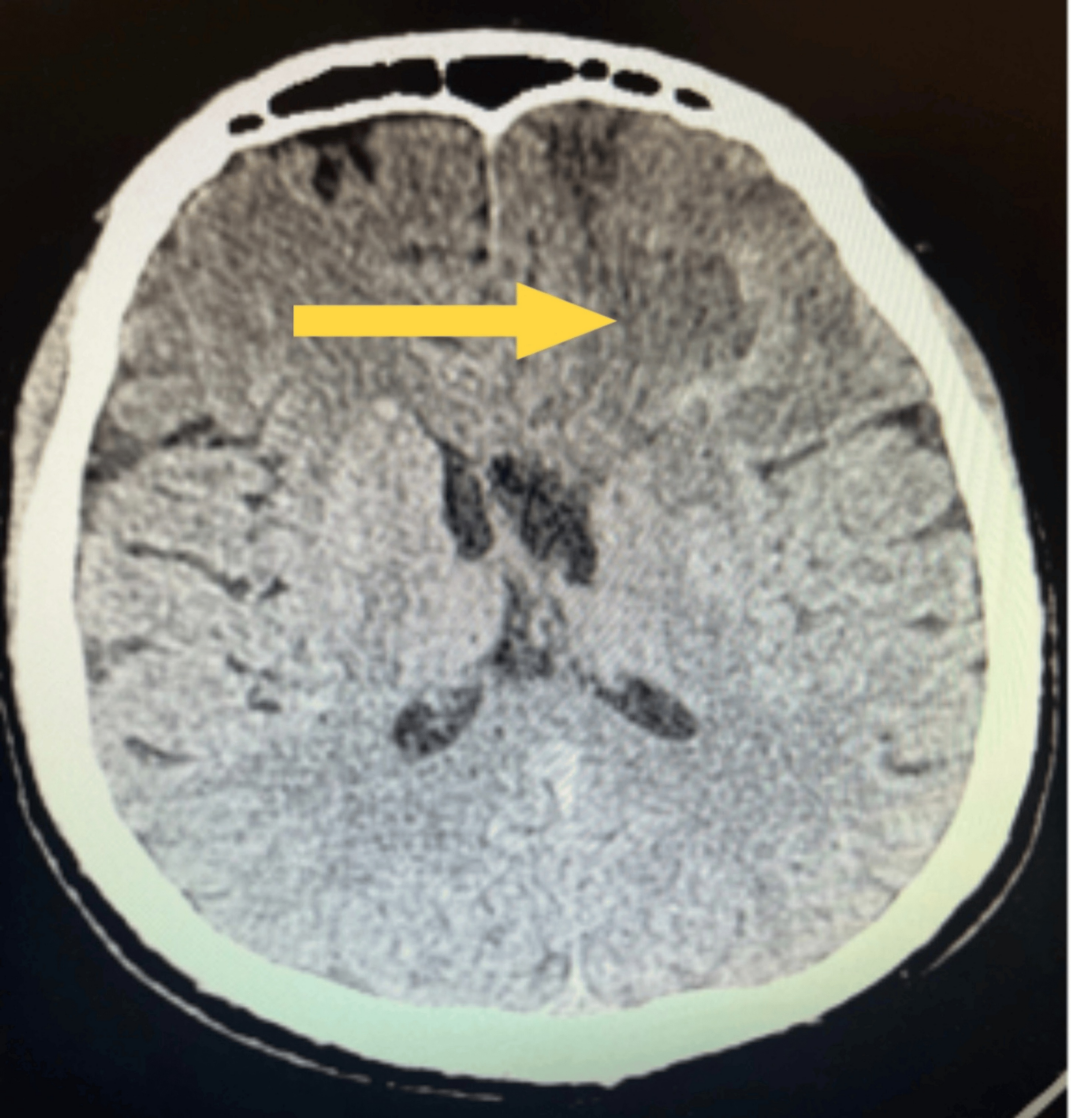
Follow-up CT scan of the head on day 22 of treatment Follow-up CT on day 22 demonstrating complete resorption of the intracerebral hematoma with cortical-subcortical atrophy and mild ventricular dilatation.
